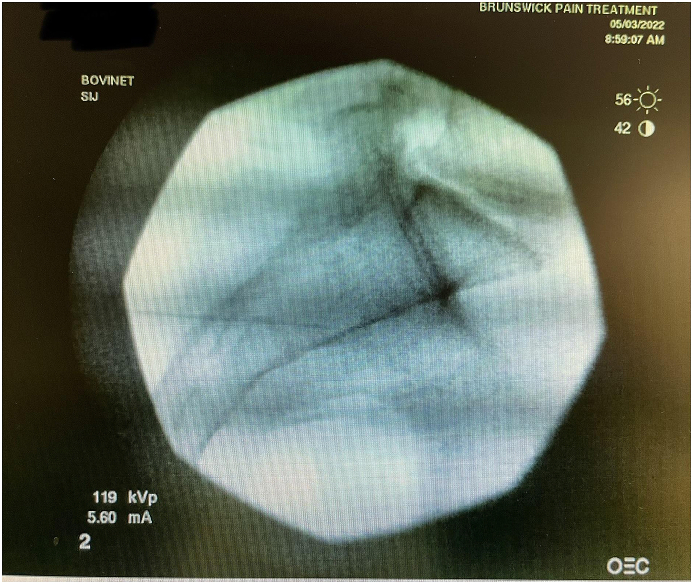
Lateral fluoroscopic image of needle trajectory. This figure depicts the needle from a diagnostic block and how the trajectory should be the same as the scout line extending across the joint on sagittal images of MRI or CT.
